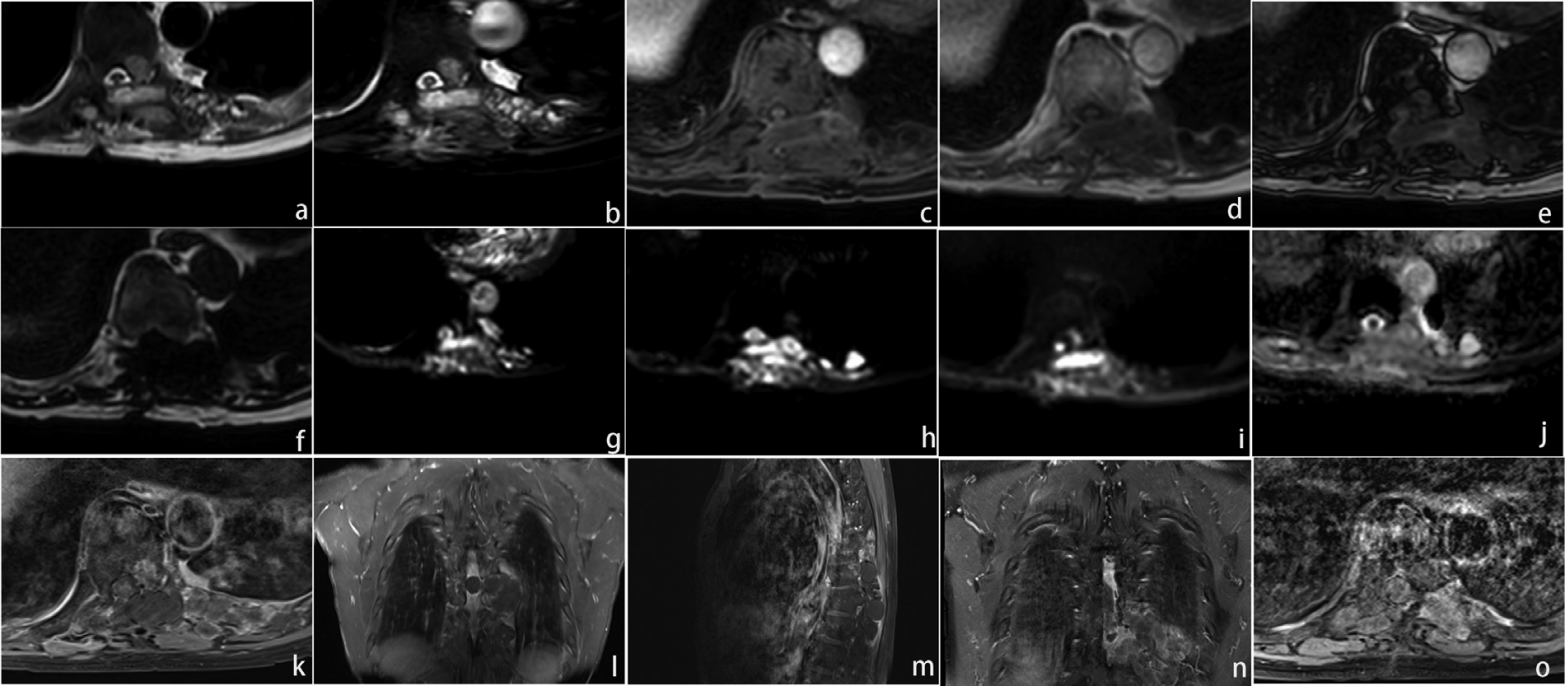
(a) MRI T2WI (axis image) showing hyperintense signal of a multilocular cystic cavity at the left side of the vertebral body. (b) MRI T2FS showing hyperintense of the cavity content. (c) TIWI Dixon sequence: WO image showing isointense signal of the lesion. (d) IP image showing hypointense. (e) OP image. (f) FO image showing hypointense. (g) MRI DWI (b = 0). (h) DWI (b = 600). (i) DWI (b = 1000). (g–i) showing hyperintense of the cavity. (j) MRI ADC map showing isointense. (k) Enhancement MRI (axis scan) showing the cystic cavity content without enhancement and the wall with slight enhancement. (l) Enhancement MRI (coronal scan) showing the cystic lesions in the spinal canal no enhancement. (m) Enhancement MRI (sagittal scan) showing the lesion ranges from T8 to T10 level. (n) Enhancement MRI (coronal scan, 2021) showing the lesion is larger, and the boundary with surrounding tissues unclear. (o) Enhancement MRI (axis scan, 2021) showing the cavity with more obvious enhancement.
